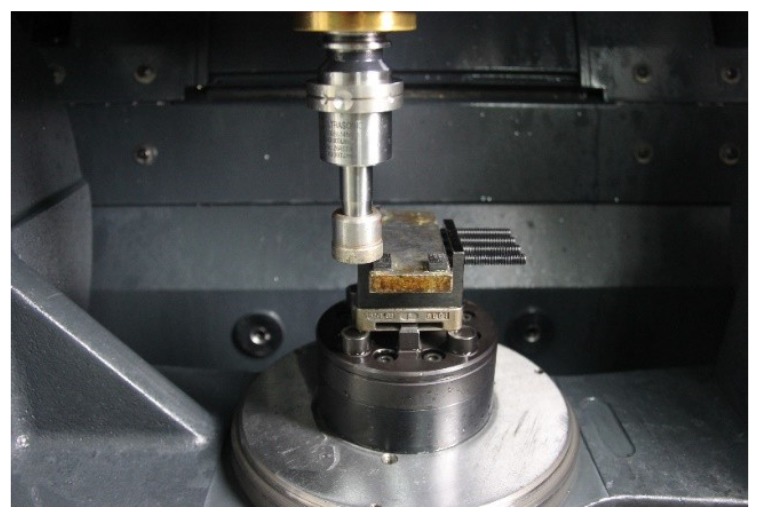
Test devices for ultrasound-assisted cutting with a single abrasive particle.
